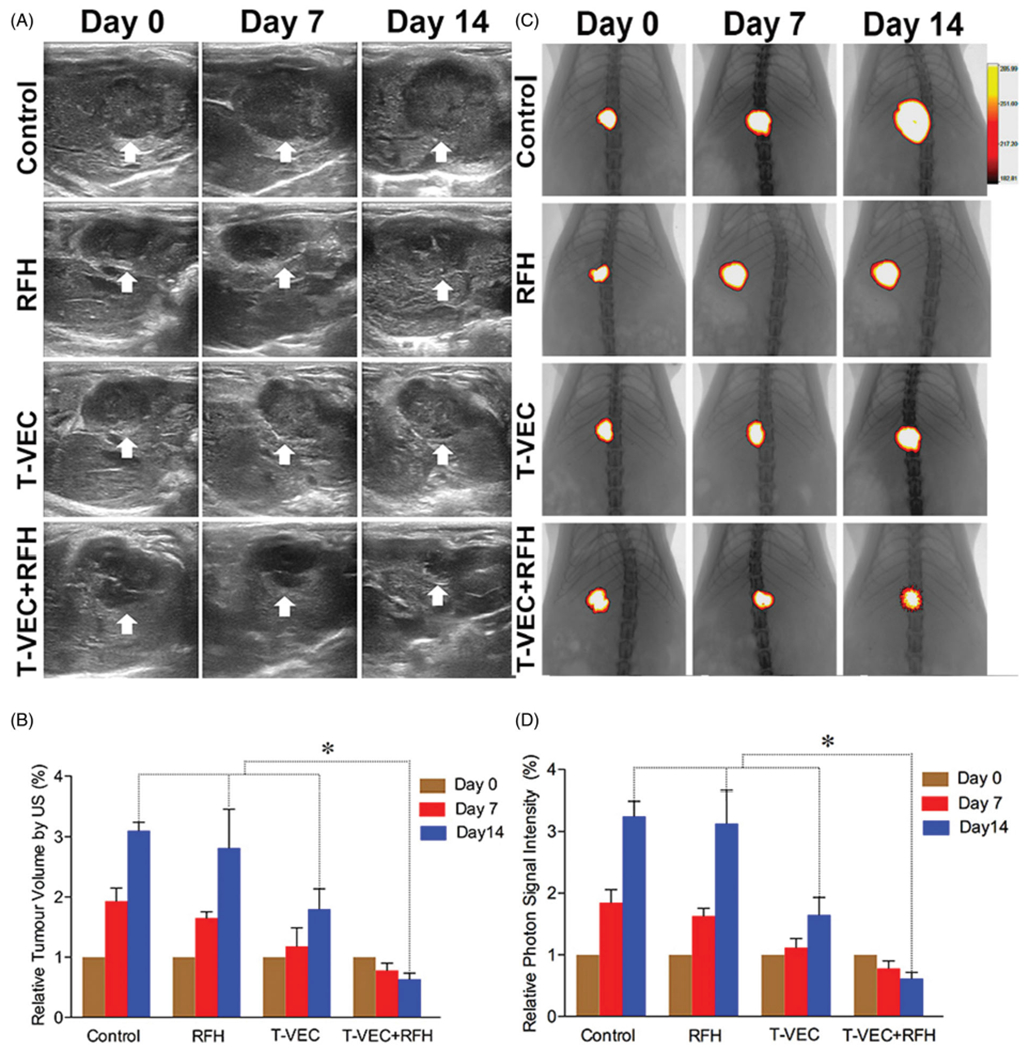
(A&B) Ultrasound imaging is used to follow up the tumor growth (arrows) at days 0, 7 and 14, showing a significant decrease in relative tumor volume with combination therapy compared with other three treatments (*n*=6/group, p*<.005). (C&D) Optical/X-ray imaging is used to follow up tumor responses to the treatments at days 0, 7 and 14, demonstrating a significant decrease in both relative fluorescent signal (golden-yellow color) and tumor size with combination therapy compared to other three treatments (*n*=6/group, p*<.05).
